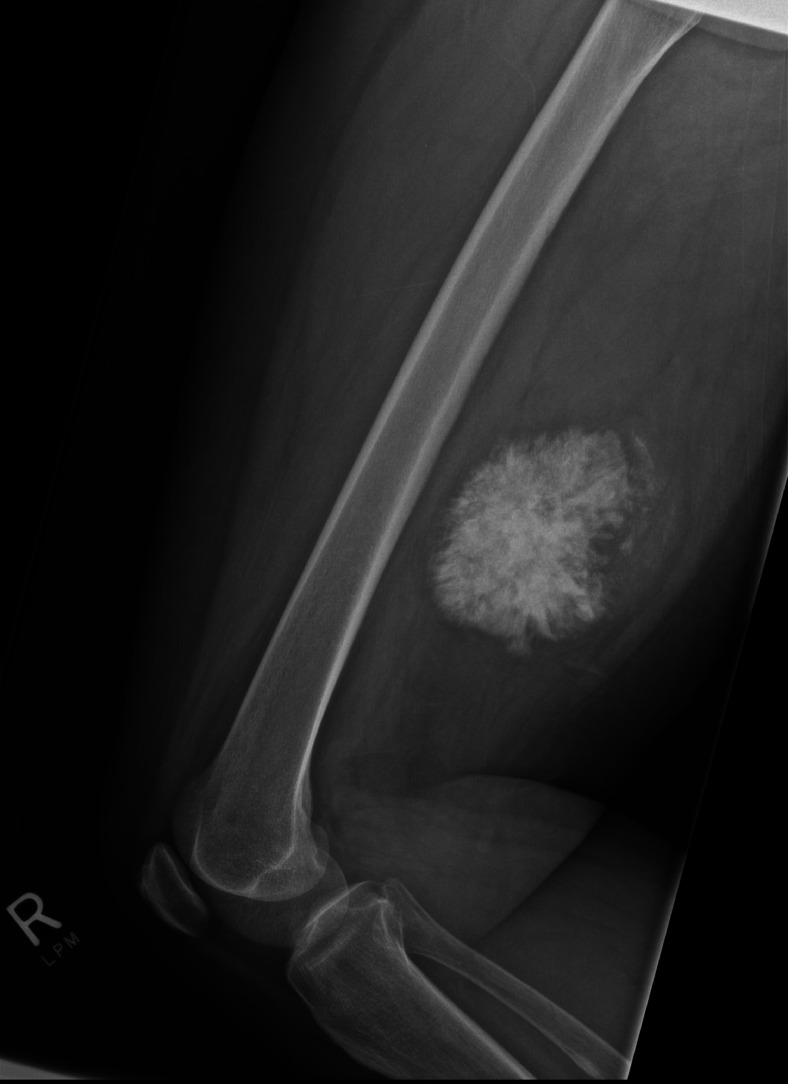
Right femur radiograph showed an extraskeletal calcified mass in the right posteromedial thigh with osteoid matrix. There are ill-defined central calcifications and margins. No involvement of the right femur. No periosteal reaction.
